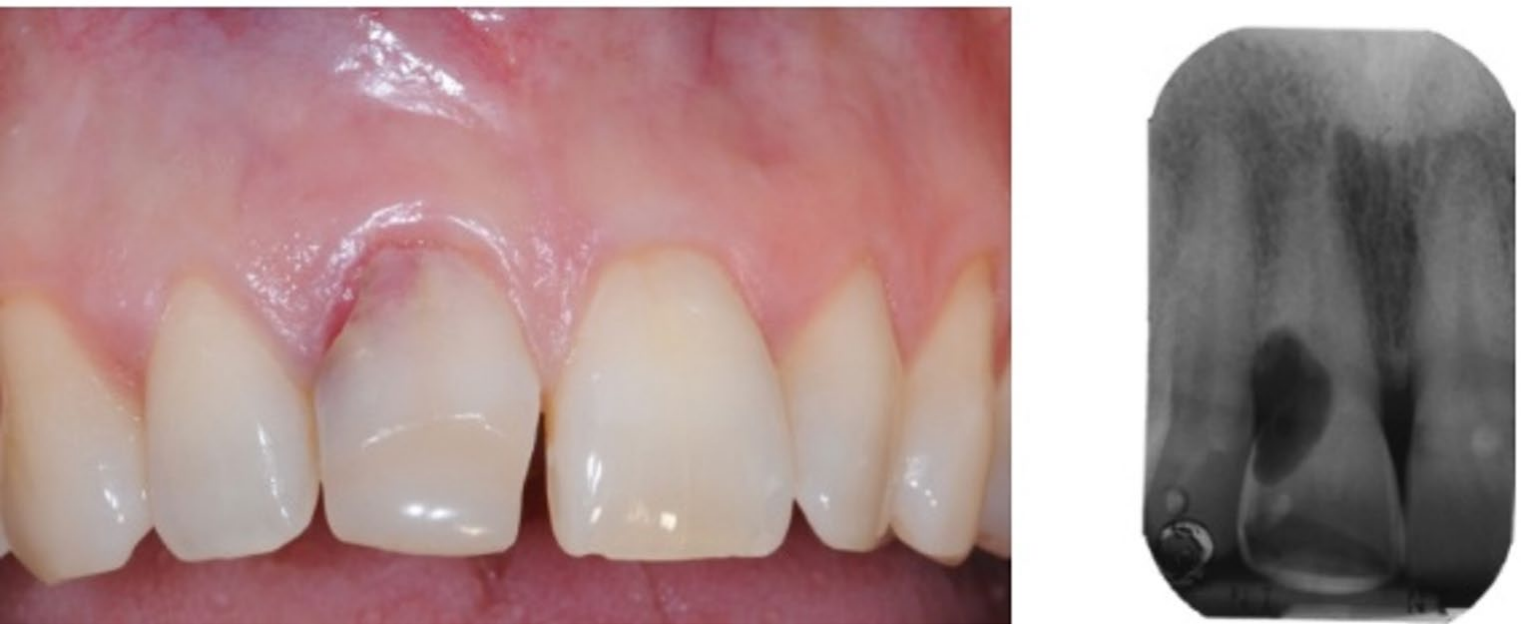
Initial condition and initial X-Ray
